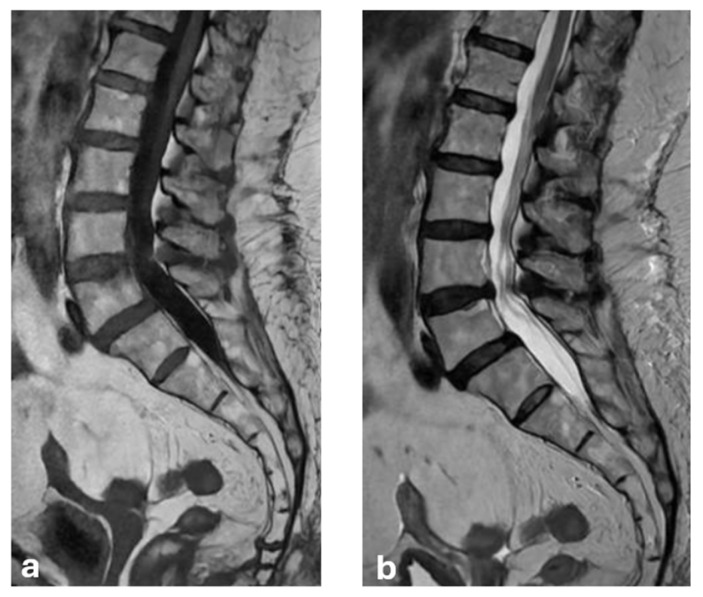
Type 1 Modic change. (a) T1-weighted. (b) T2-weighted.
